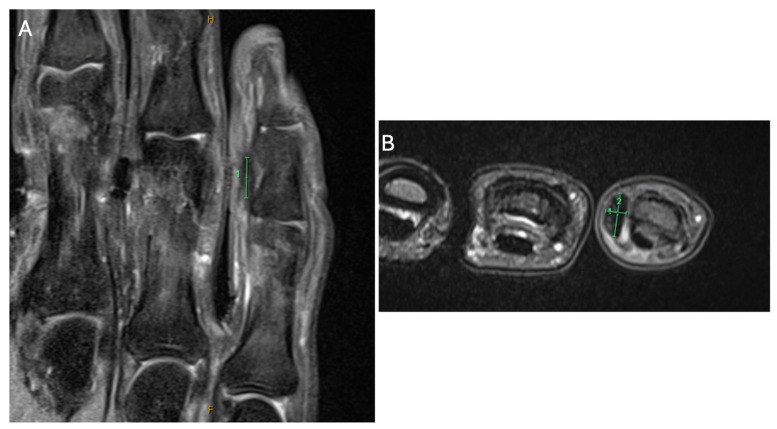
Magnetic resonance imaging (MRI) of the right little finger. (A) Coronal MRI demonstrating the longitudinal extent of the lesion along the digit. (B) Axial MRI section of the right little finger demonstrating the lesion in cross-section.
